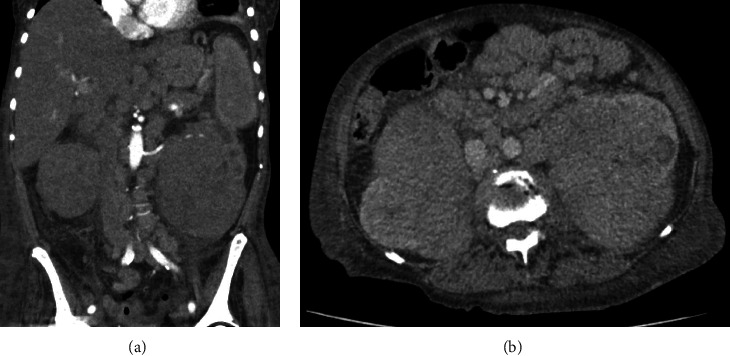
CT scan showing renal/pararenal masses in the patient. (a) Abdominal coronal plane, showing masses with near-total compression/infiltration of the kidneys. Remaining renal structures of the left kidney are visible in this section. (b) Transverse plane of the same patient.
